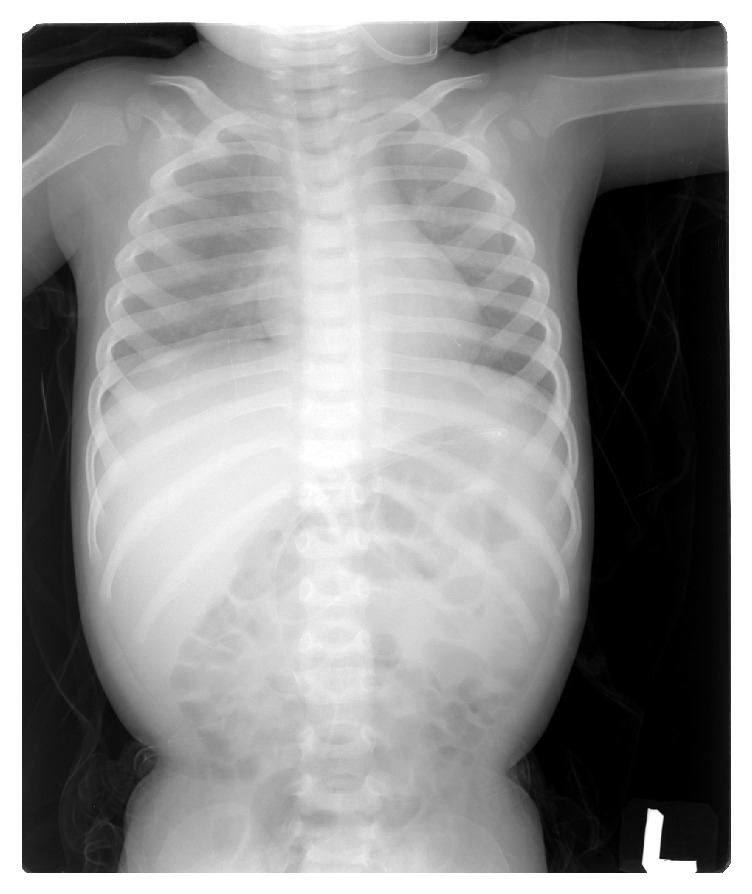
X-ray: the previous multiple bony fractures with callus formation are not prominent on the following plain film radiograph 6 months later.
